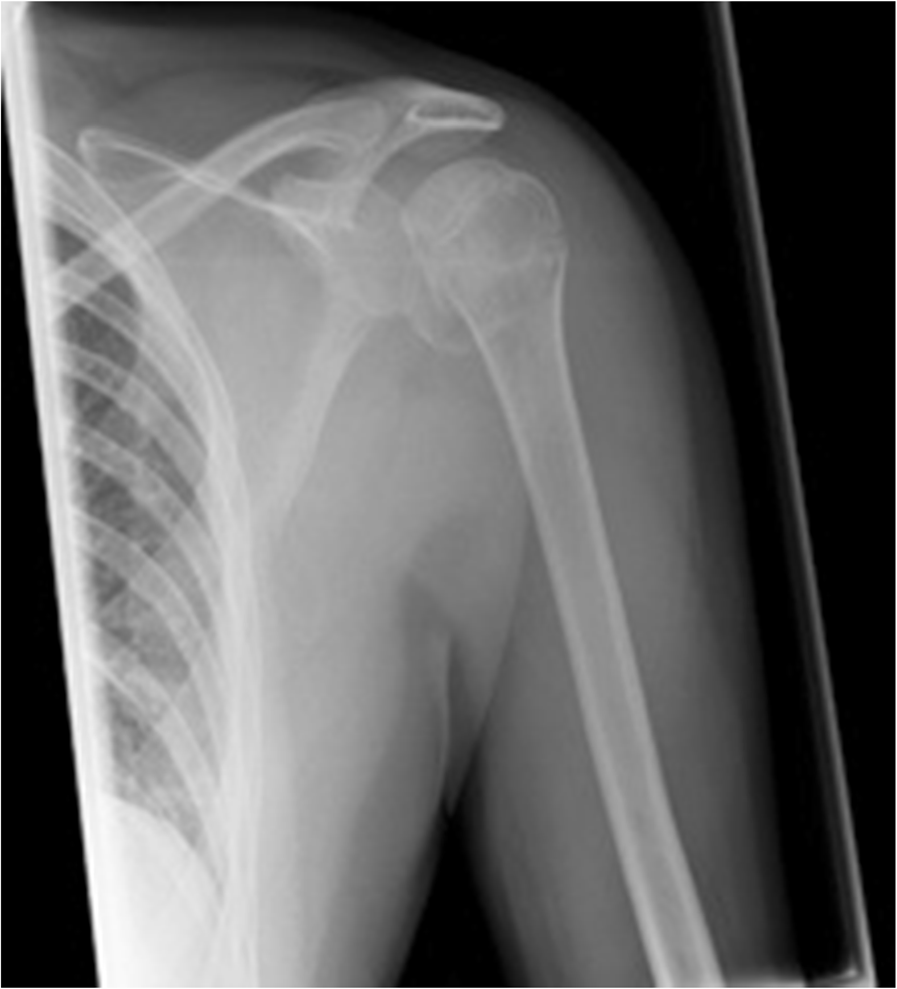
Pre-operative x-ray showing the posterior dislocation and the reverse Hill-Sachs lesion
